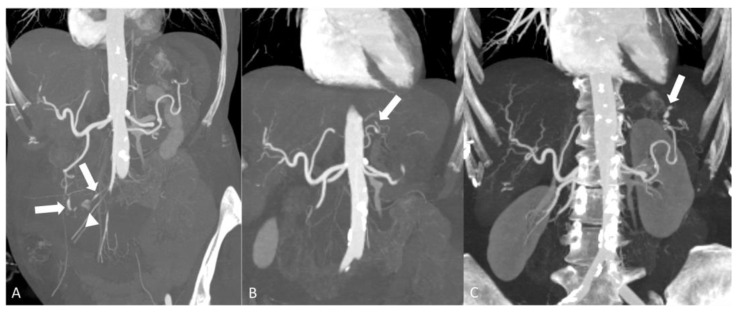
An 80-year-old woman with polyarteritis nodosa presenting with gastrointestinal bleeding. Coronal maximum intensity projection images of abdomen CTA at three different levels demonstrating pseudoaneurysms of the celiac and superior mesenteric artery branches secondary to arteritis. (A) Pseudoaneurysm of the gastroduodenal artery and jejunal branch of the superior mesenteric artery (arrow) and contrast extravasation into the proximal small bowel loop consistent with active GI bleed (arrowhead). (B) Pseudoaneurysm of the left gastric artery (arrow). (C) Pseudoaneurysm of the distal aspect of the splenic artery (arrow).
